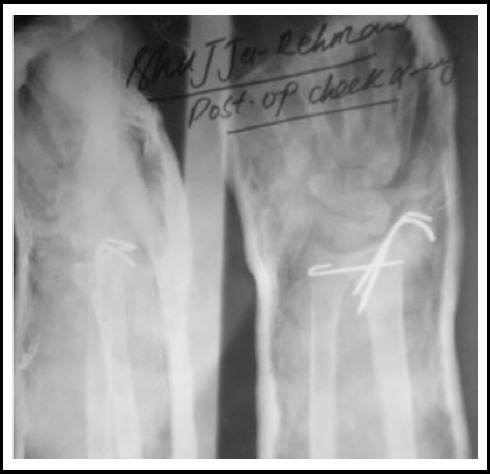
Post Operative Radiograph with AP & Lat View.
